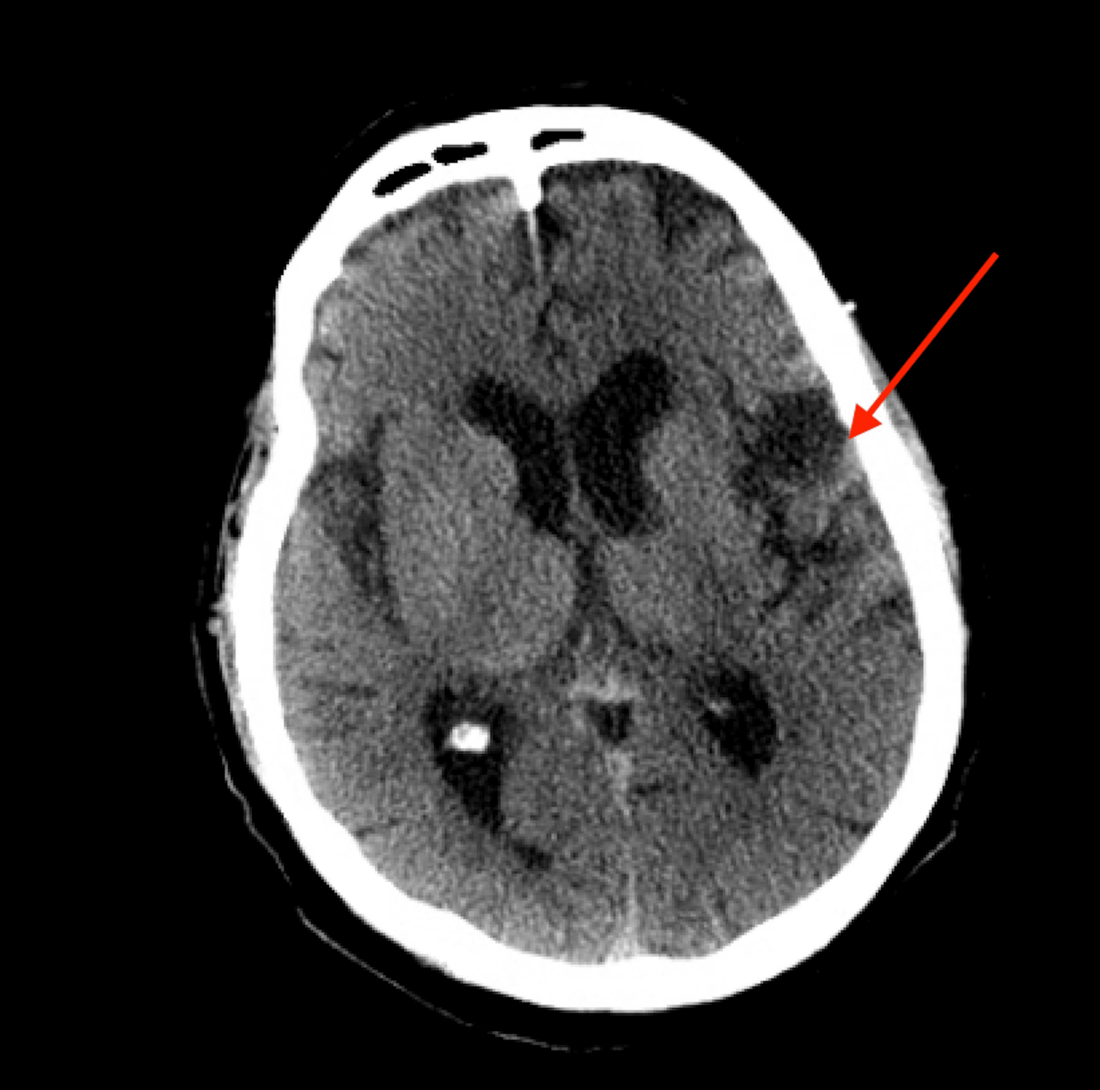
CT head showing left parietal lobe encephalomalacia CT: computed tomography
